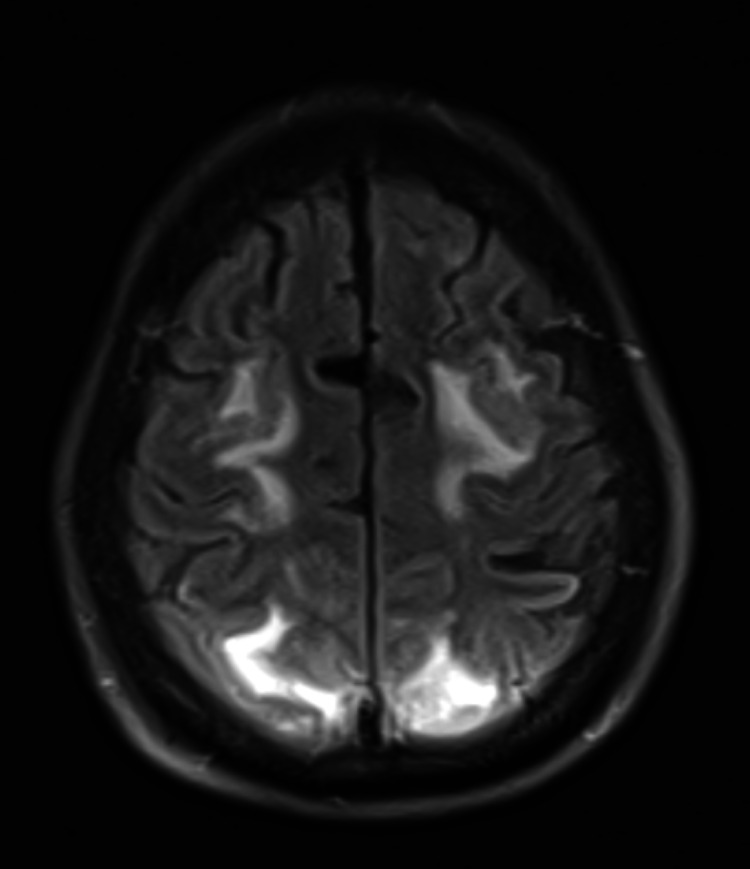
T2/FLAIR hyperintensities of bilateral ACA and PCA territories. FLAIR: fluid-attenuated inversion recovery; ACA: anterior cerebral artery; PCA: posterior cerebral artery
